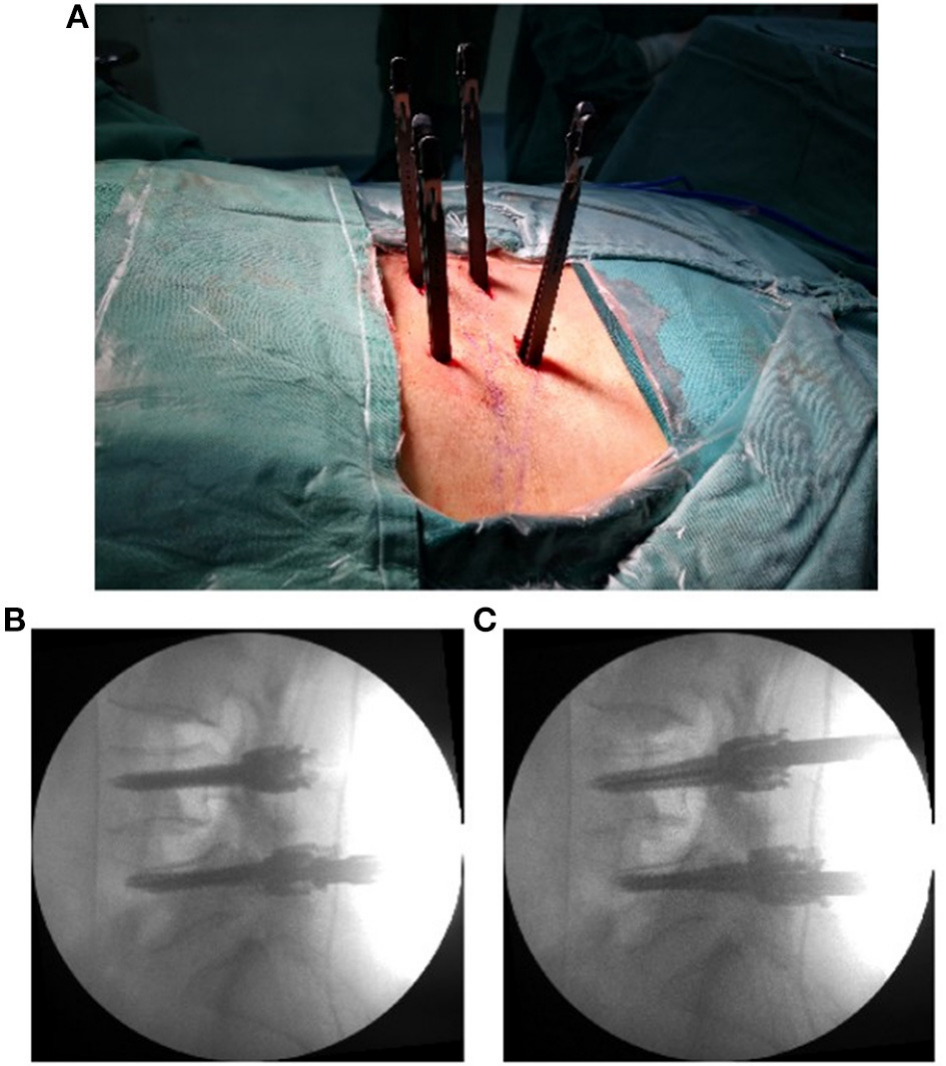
The pedicle screws were placed percutaneously (A). The positions of the screws are shown in the X-ray lateral view (B), and the intervertebral foramen was enlarged by a pedicle screw distractor (C).
